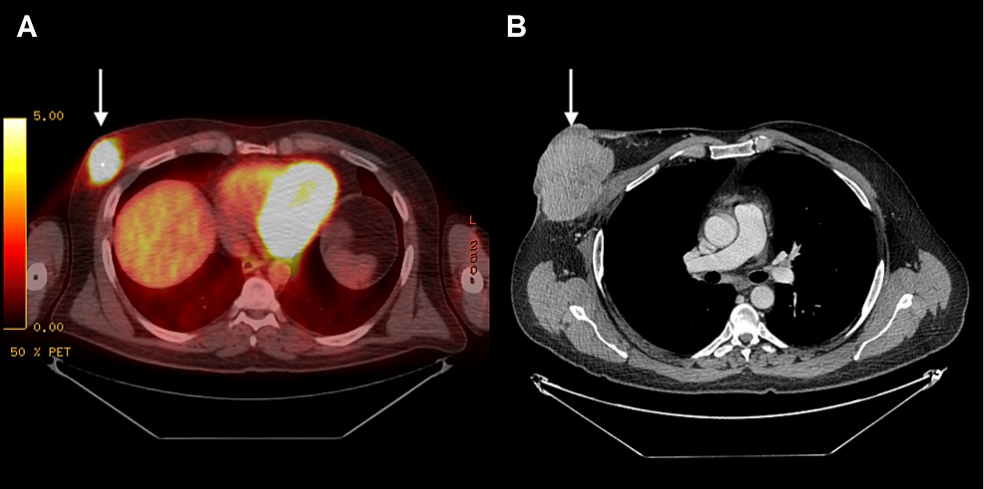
FDG-PET/CT and contrast-enhanced chest CT. (A) FDG-PET/CT shows intense hypermetabolic activity at the lobulated right chest wall mass with an SUV max of 7.4. No FDG-avid nodal or metastatic disease is present. (B) The eight-month follow-up contrast-enhanced chest CT demonstrates a marked increase in the size of the known right chest wall mass. The mass now exhibits areas of internal hypoattenuation and has invaded the pectoralis major. FDG-PET/CT: fluorodeoxyglucose positron emission tomography-computed tomography; SUV: standardized uptake value.
